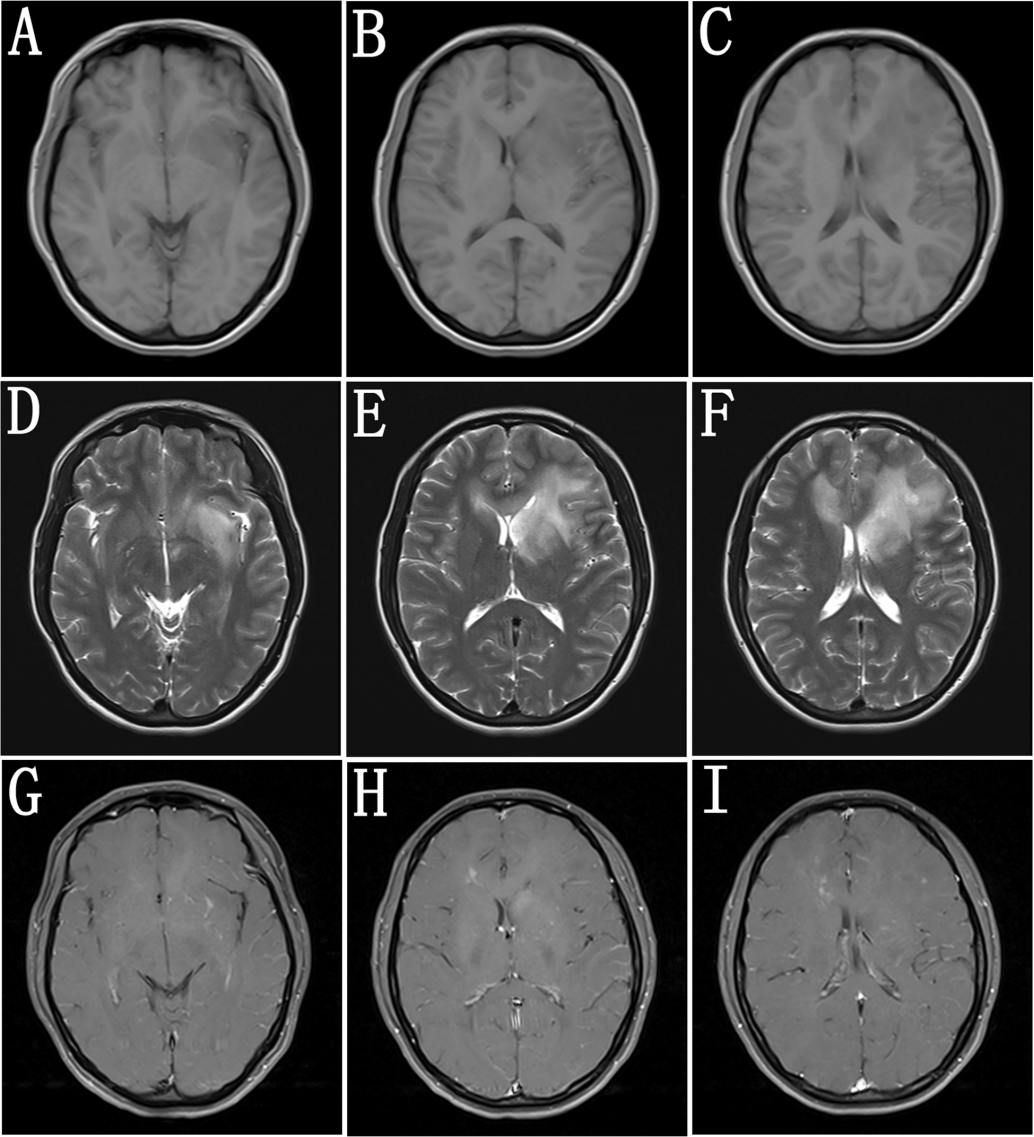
Brain MRI before treatments. Ten days after symptom onset, brain MRI revealed diffuse lesions in the left temporal and frontal lobes, the left basal ganglia area, the left splenium of corpus callosum, and the right frontal lobe, which were hypointensity on T1WI (a-c), and hyperintensity on T2WI (d-f). The lesion in the left basal ganglia area had mild edema and the anterior of left lateral ventricule was compressed (b, e, h). Barely contrasted lesions were present in the above lesions (g-i)
